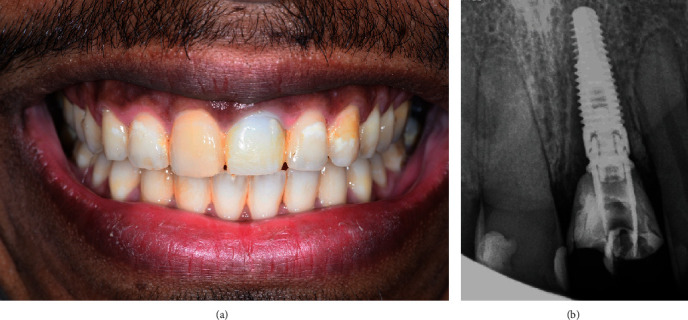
Clinical and radiographic presentation of the immediately placed implant with provisional restoration for tooth #21. (a) Intraoral frontal view. (b) Periapical radiograph.
